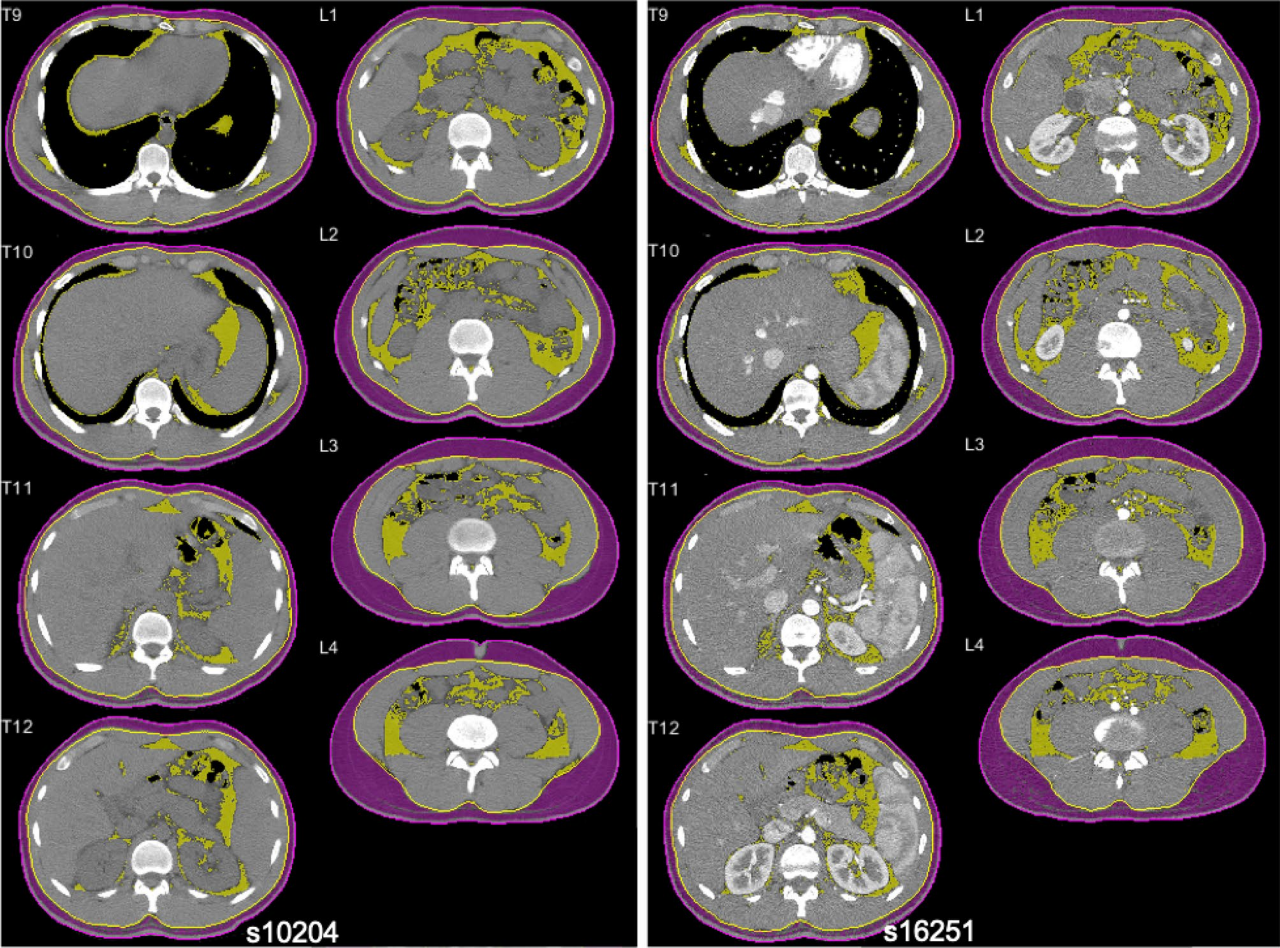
Example of healthy 20 y/o male non-contrast (left) and contrast (right) CT axial slices, showing T10-L4 visceral fat area (yellow-shaded region), subcutaneous fat area (purple shaded region), outer abdominal fascia boundary (yellow line), and skin boundary (purple line). Portions of skin boundary that are coincident with the scan field of view are highlighted in red.
